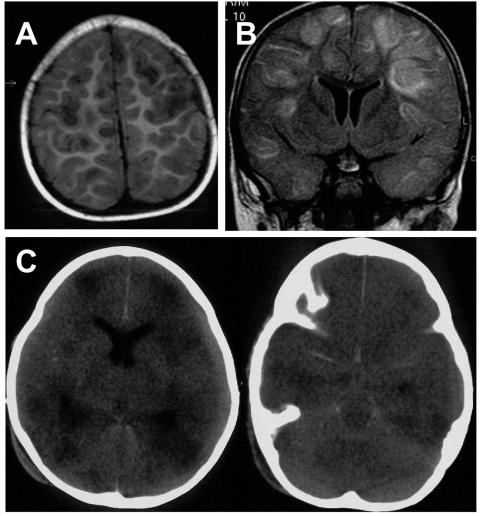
Axial T1-weighted magnetic resonance imaging (MRI) scan (A) and coronal fluid attenuated inversion recovery (FLAIR) (B) show multifocal, mainly cortical and subcortical lesions of high signal intensity, which are most probably caused by multifocal encephalitis. C) Nonenhanced axial computed tomographic (CT) scan performed 2 days after the MRI shows multiple, hypodense lesions and signs of general edema. Additionally, it shows a hyperdense arachnoid collection that was not yet visible on the MRI 2 days before (panels A and B).
